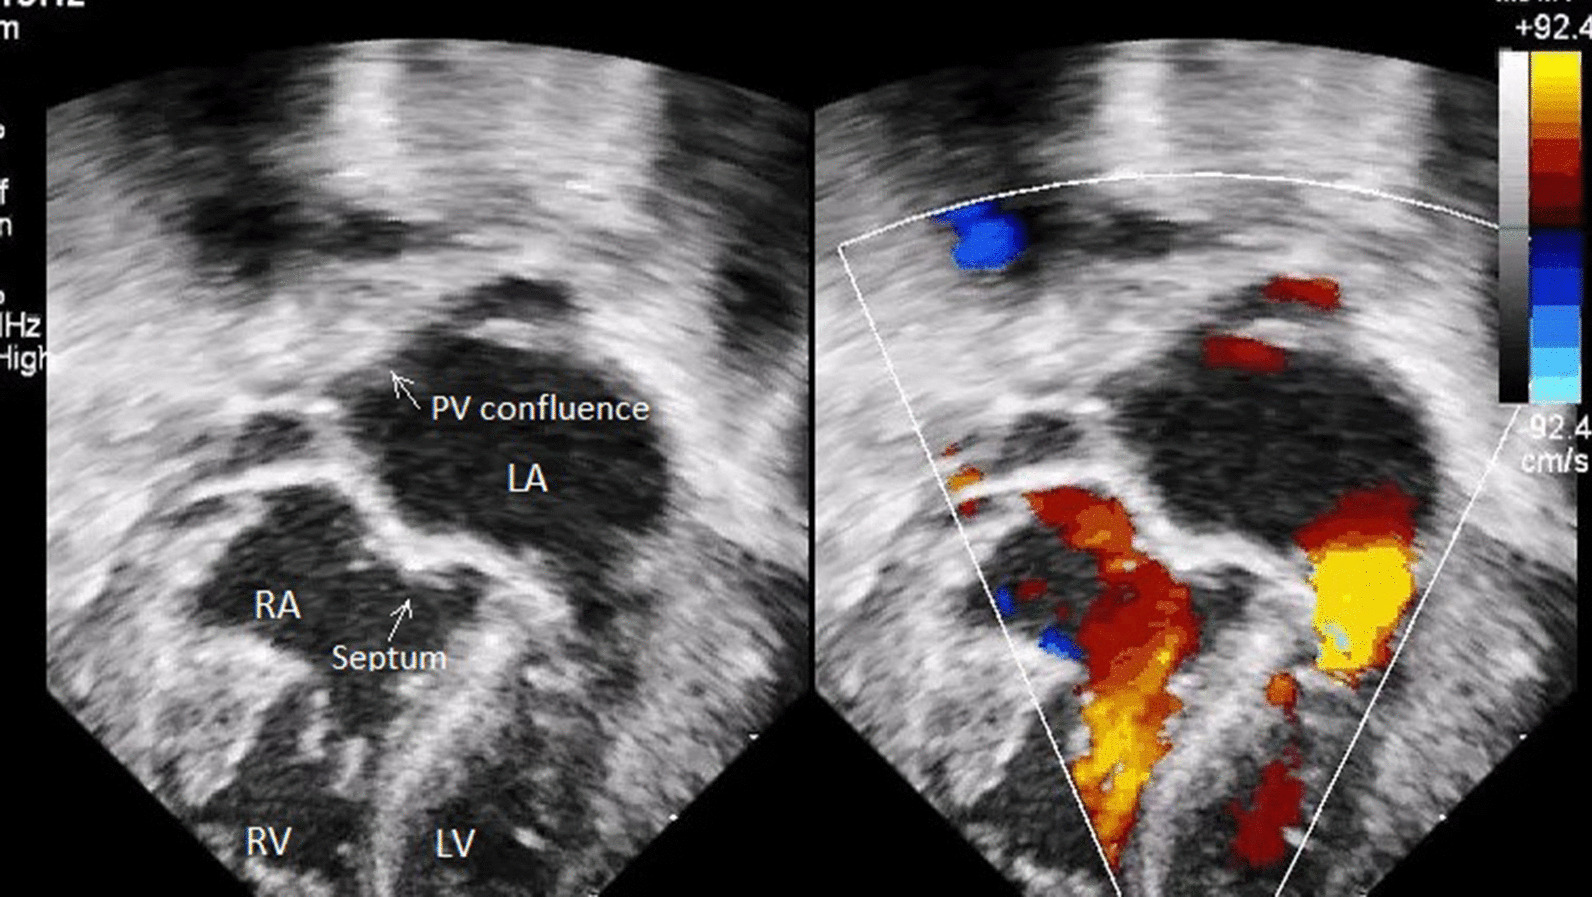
Postoperative 2-D echocardiogram including colour Doppler showing partition of atrium and diversion of pulmonary venous drainage into the left atrium (LA—left atrium, RA—right atrium, RV—right ventricle, LV—left ventricle, PV—pulmonary veins)
